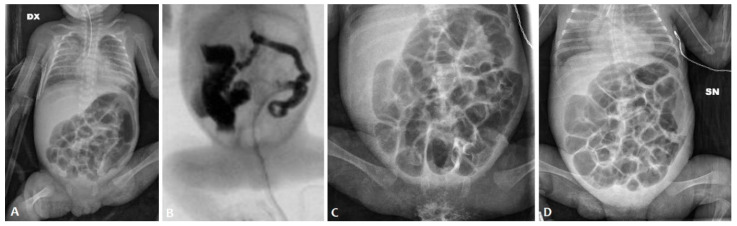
Abdominal X-ray of patient P1 on the 10th day of life (A); contrast enema performed on the 26th day of life (B); abdominal X-ray before the NAC treatment (C) and after the NAC treatment (D).
